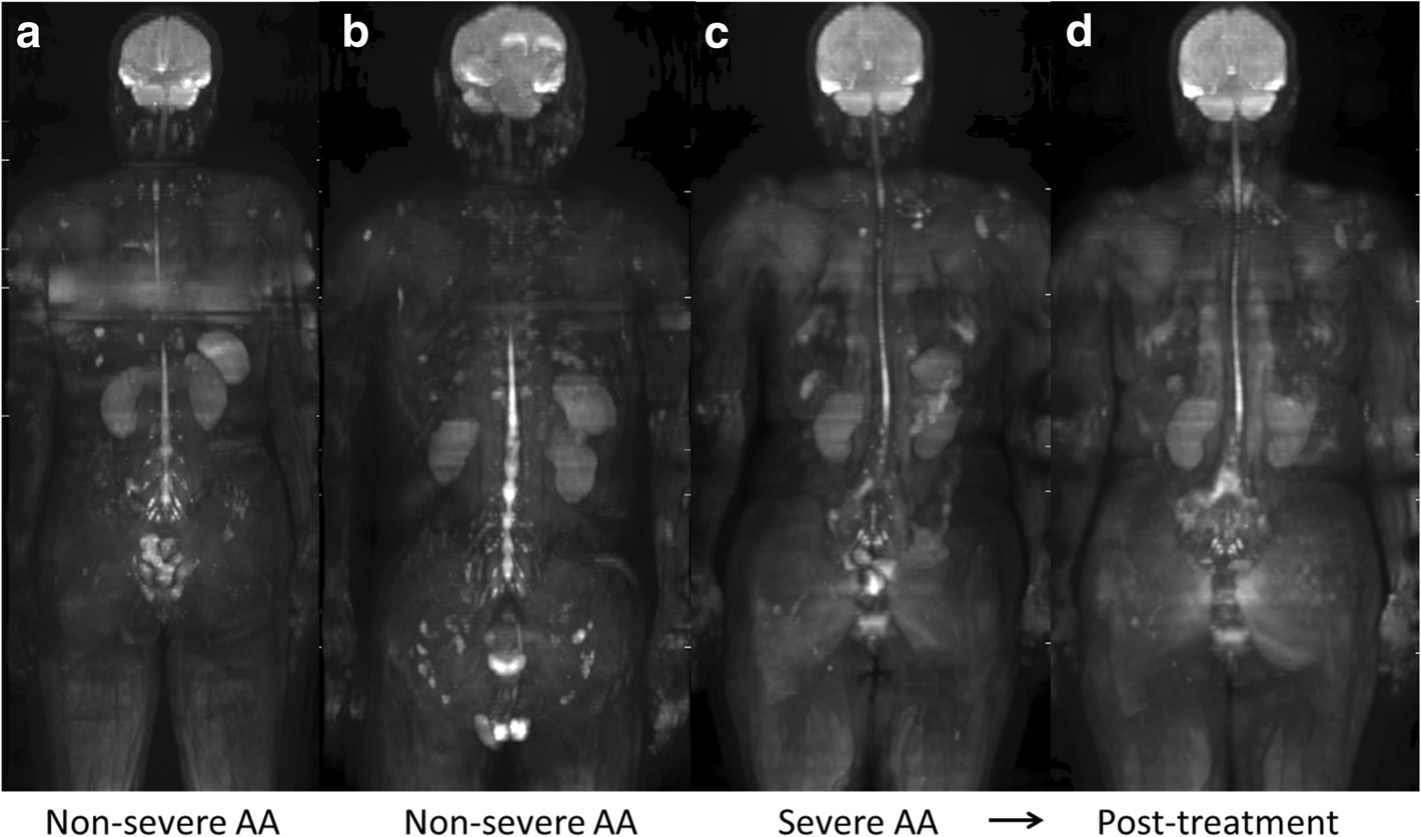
Maximum intensity projection DWI images of AA patients (a–d). a–d in Fig. 4 are identical to a–d in Fig. 3, respectively. Whole-body DWI did not demonstrate any significant differences in bone marrow signals between non-severe AA and severe AA patients, or between pre- and post-treatment scans
